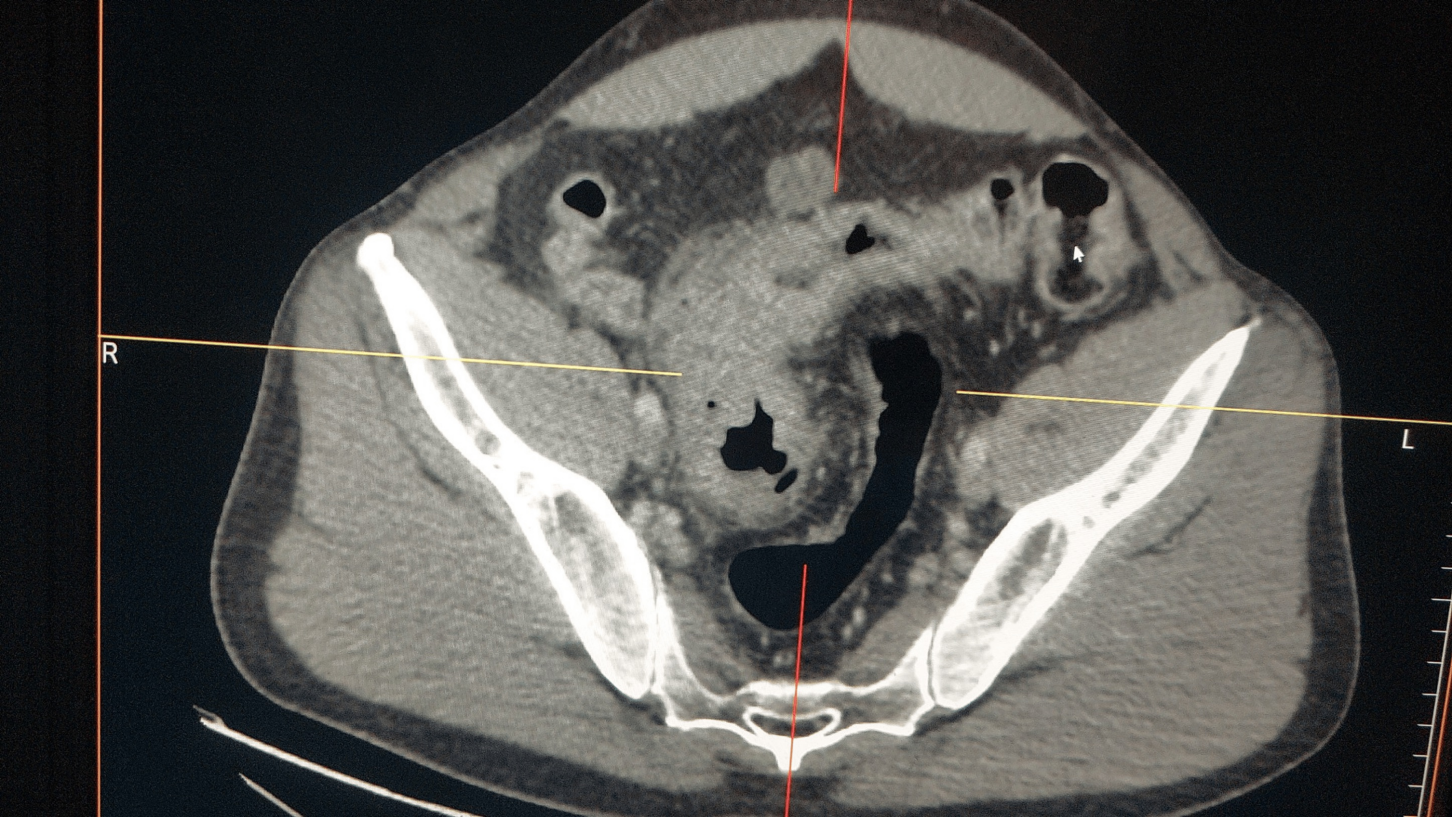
Computed tomography scan revealing an adenocarcinoma of the colon
